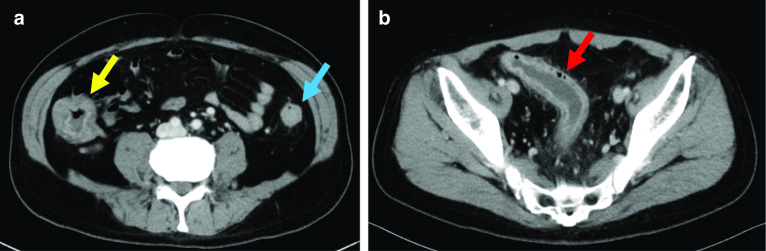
CT showed edematous and thickening of the colon [a ascending colon (yellow arrow) and descending colon (blue arrow), and b sigmoid colon (red arrow)]
